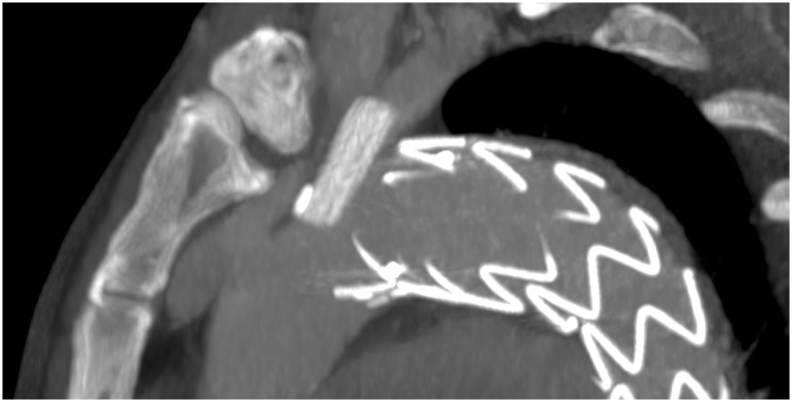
Post-operative CT-angiography after the first procedure showing the good positioning of Thoracic graft and stent in left subclavian artery.
